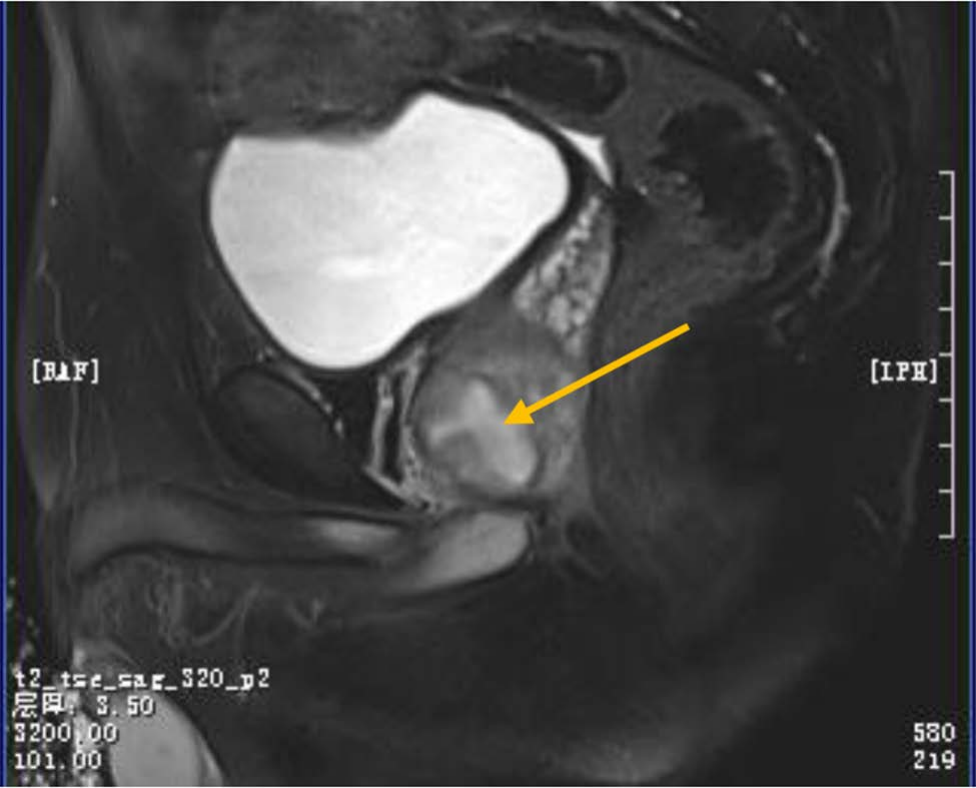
Magnetic resonance imaging of the prostate showed a prostate abscess.
